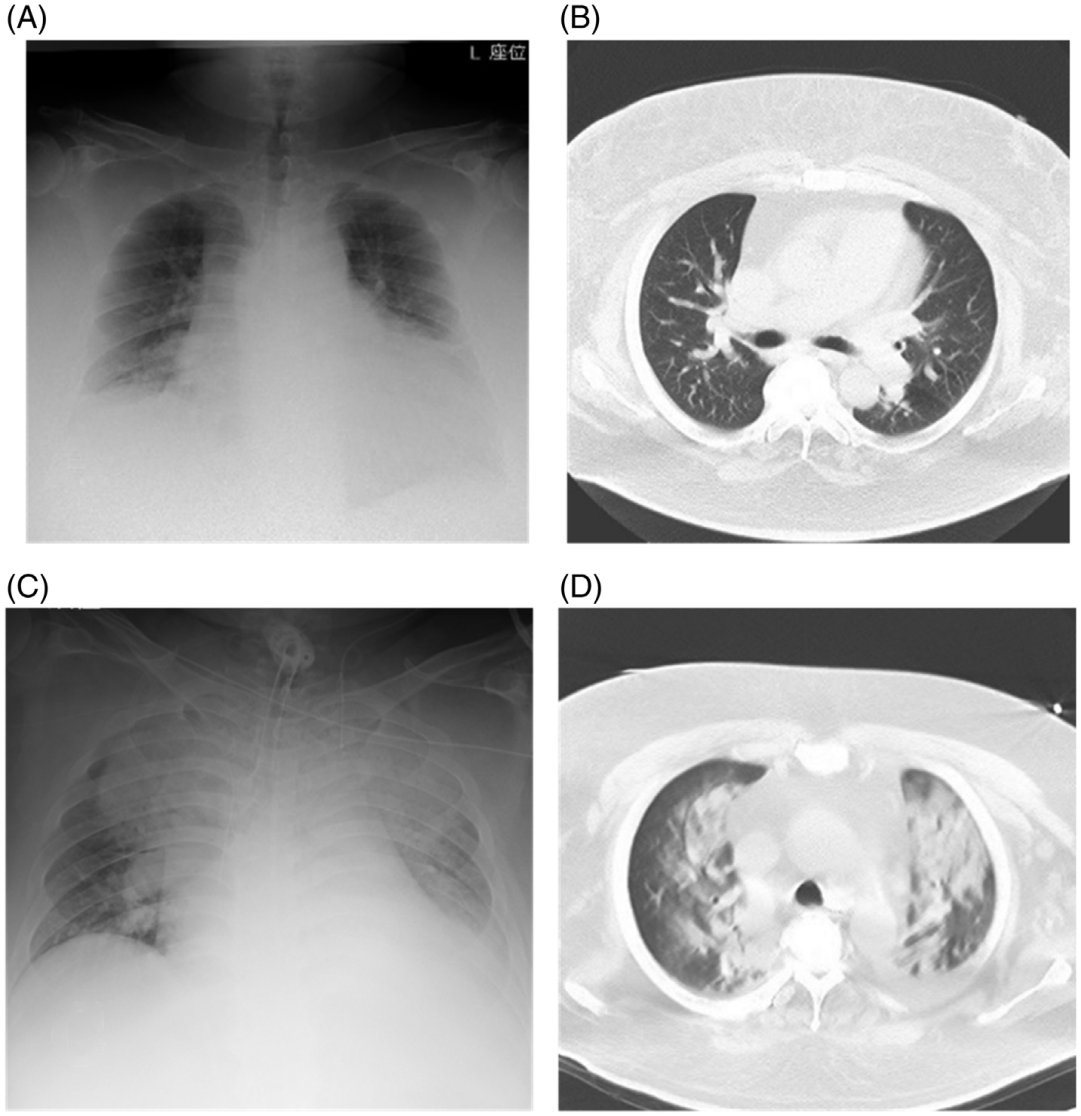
Chest X‐ray and CT of case 2 (A) Chest X‐ray on admission showed an increased cardiothoracic ratio. (B) CT on admission revealed thick subcutaneous fat surrounding the thorax and no infiltration in the pulmonary fields. (C) Chest X‐ray on day 11 showed extensively increased density in the bilateral lungs due to acute respiratory distress syndrome. (D) CT on day 11 revealed extensive infiltration in the pulmonary fields
